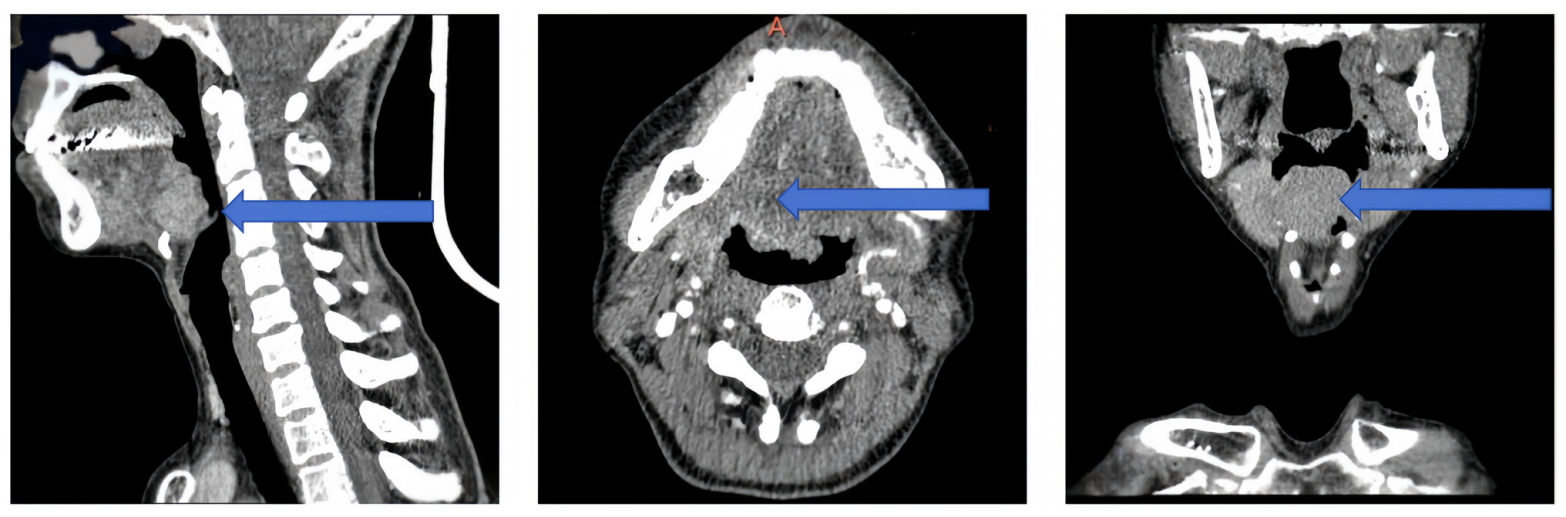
Preoperative enhanced CT images of the patient. The sagittal, horizontal and coronal planes of the CT images reveal a well-defined nodular soft tissue shadow on the right side of the tongue root (arrow). The lesion had regular edges, although the tongue border was unclear. The maximum cross-section measured ~3.7×2.3 cm, with no enlarged lymph nodes in the maxillofacial and cervical regions. Additionally, there was no apparent thickening or protrusion on either side of the inner wall of the vocal folds. The laryngeal soft tissue structure remained symmetrical, with no apparent high-density masses. The epiglottis was not visibly enlarged, the pyriform sinuses on both sides and the paralaryngeal space were clear, and there was no damage to the surrounding bone. CT, computed tomography.
